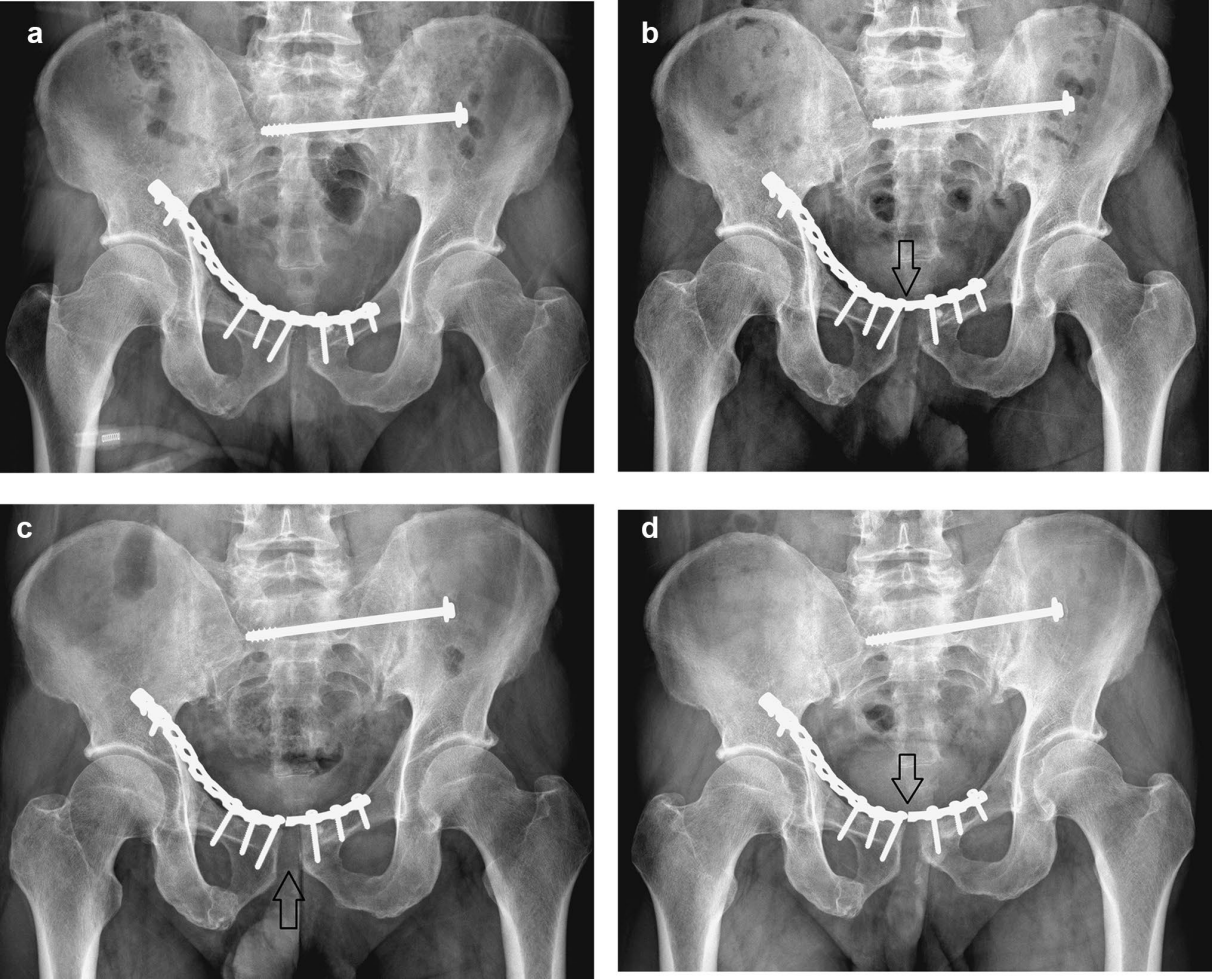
Radiographs of the pelvis in an anteroposterior view after anterior plating and posterior screw fixation. a Immediately postoperative. b At 3 months, symphysis plate breakage was observed (seen at arrow). c At 6 months, the pubic symphysis distance mildly widened (arrow). d At 12 months, the pubic symphysis distance became stable without an interval change
